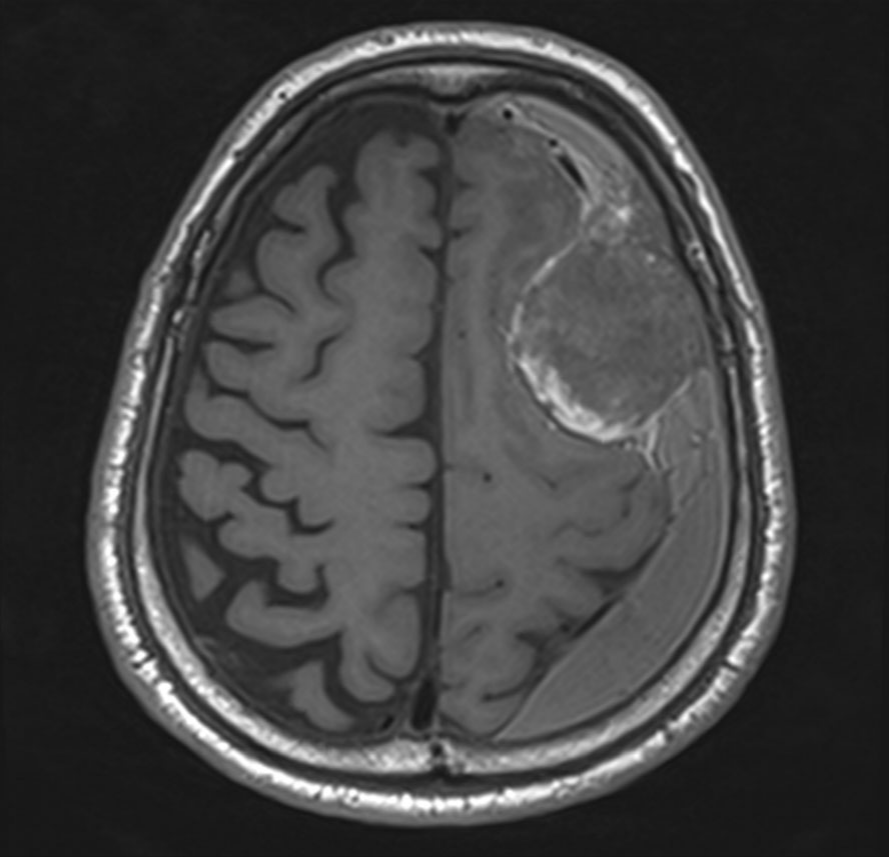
Postoperative brain magnetic resonance imaging showing intracranial metastasis 2 months after surgery.
